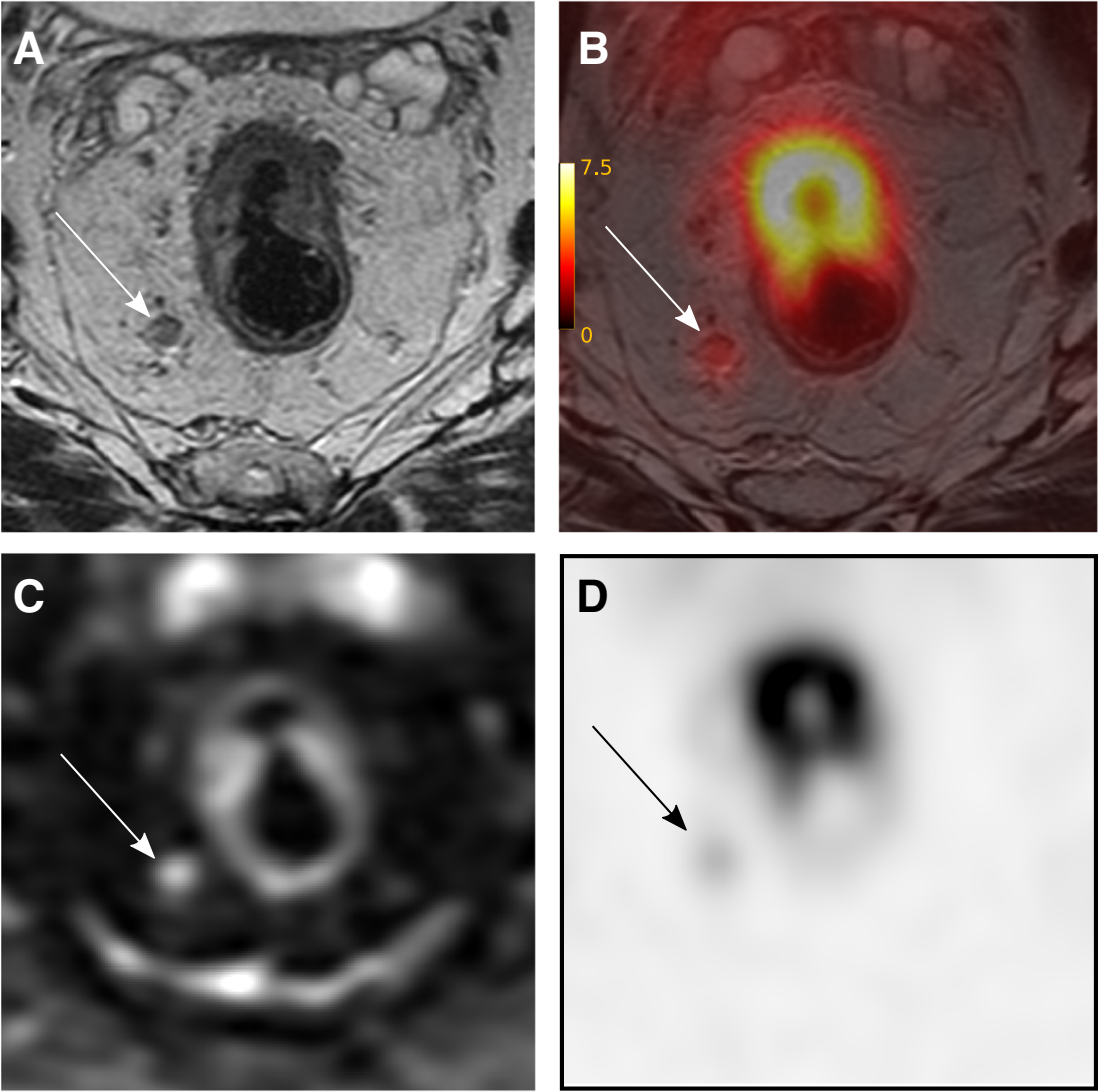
A PET/MR image of the same patient as that in Fig. 3. a Transaxial T2 weighted sequence perpendicular to the tumour; b FDG-PET/MR image with a T2 weighted MR sequence; c Transaxial diffusion-weighted sequence (b = 800 s/mm2) d Static 3D MAC PET image
